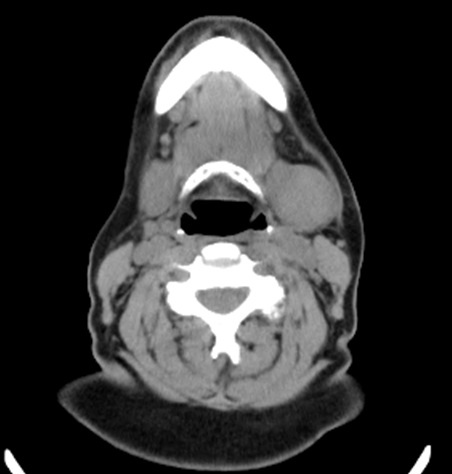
Axial computed tomography without contrast demonstrating a non-homogenous 4.5 x 3.0 x 3.0 cm mass in the left submandibular gland.
